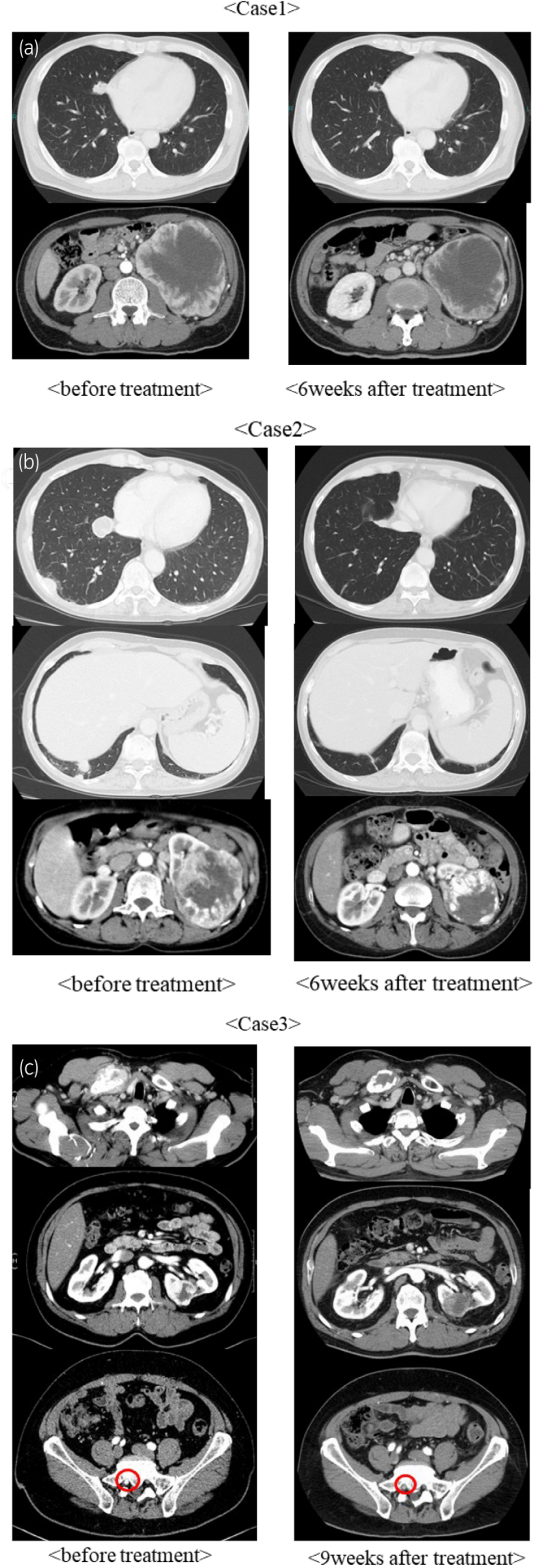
(a) Contrast CT of case 1 before and after the start of treatment. The primary tumor in the left kidney and metastases in the right lung are shown. The primary tumor did not shrink significantly, but the lung metastases were reduced. (b) CECT of case 2 before and after the start of treatment. The primary tumor in the left kidney and metastases in the right lung and pleura are shown. The lungs had multiple metastases. The treatment resulted in shrinkage of the tumor, and most of the lung metastases disappeared. (C) CECT before and after the start of treatment in case 3, showing the primary tumor in the left kidney and metastases in the left clavicle, left scapula, and sacrum. The sacral metastases are located near the neural foramen, circled in red. Posttreatment, the scapular and sacral metastases had disappeared. The kidney and clavicle metastases remained the same size, but the internal contrast effect was diminished.
